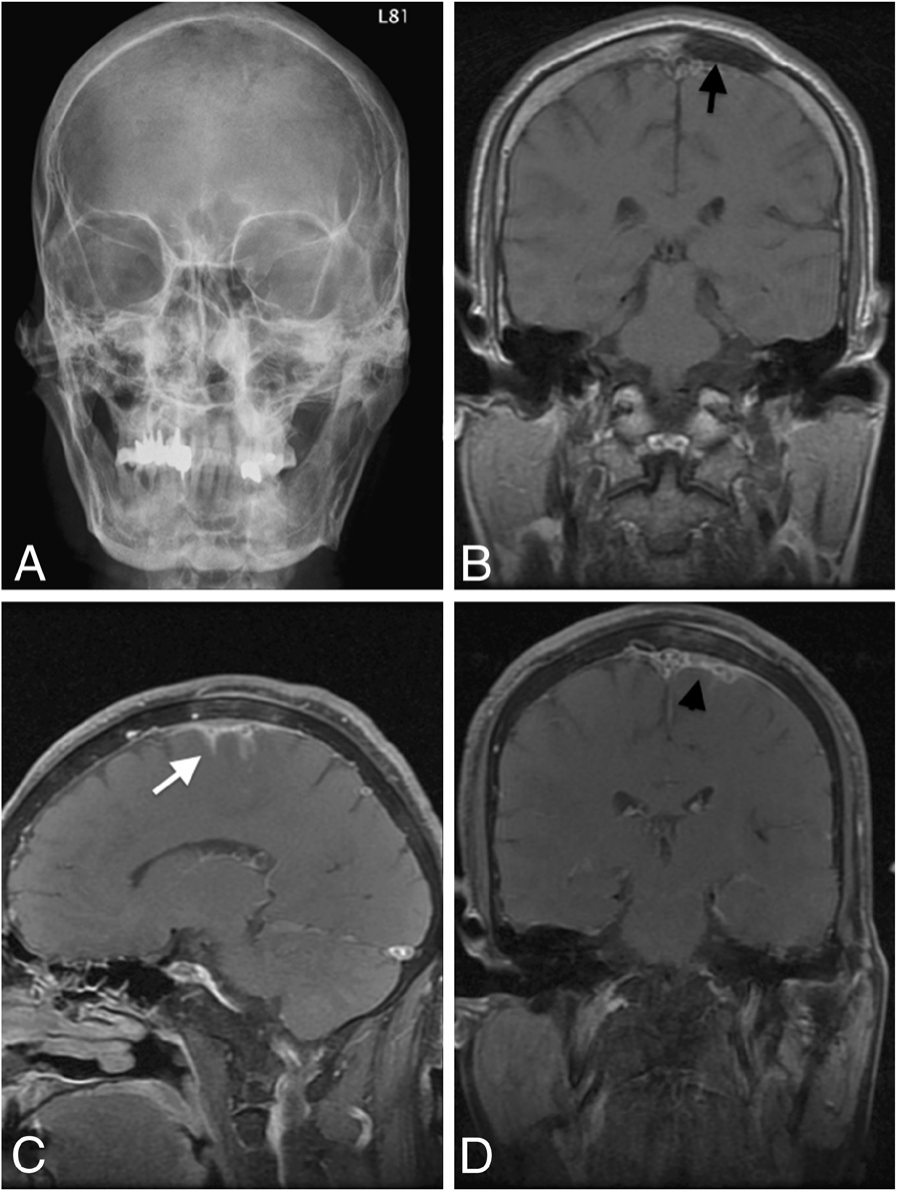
Preoperative images. A. Anteroposterior view of skull X-ray radiography shows left skull bone effacement near the vertex. B-D. Magnetic resonance image shows an area of abnormal bony marrow over left high convexity showing loss of normal fatty marrow with moderate enhancement after contrast injection (arrow). Mild subperiosteal soft tissue thickening and adjacent dura and leptomeningeal thickening and enhancement (arrowhead) are apparent. The left central and precentral sulci are filled with abnormal enhancing lesion (white arrows).
